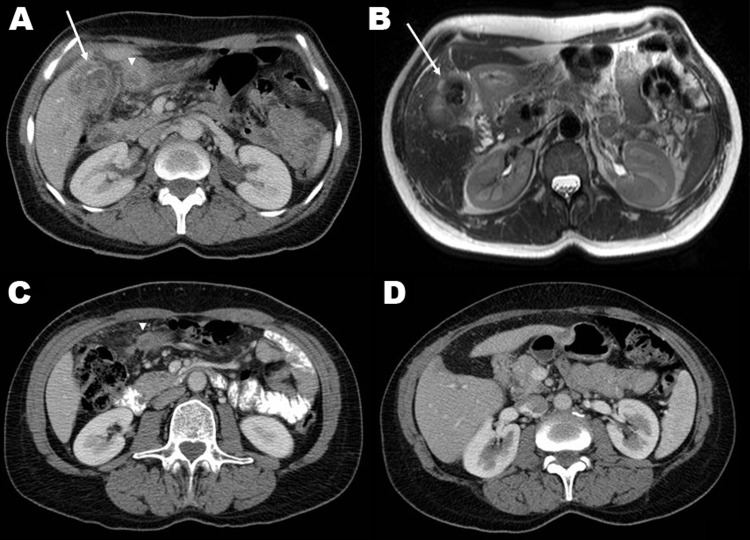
A) Contrast-enhanced computerized tomography (CT) scan showing a calcified gallbladder wall (arrow), a surrounding, calcified mass located peripherally in the liver, and an abscess in the adjacent fat tissue (arrowhead). B) T2-weighted axial magnetic resonance imaging shows multiple gallstones and a thickened gallbladder wall (arrow), inflammation and edema of the adjacent liver, fat tissue, and proximal duodenum. C) Eight weeks after cholecystectomy, contrast-enhanced CT shows a residual abscess in the adjacent fat tissue (arrowhead). D) Contrast-enhanced CT 5 months after cholecystectomy shows only minimal changes in the gallbladder bed and surrounding tissues, and no residual abscess.
